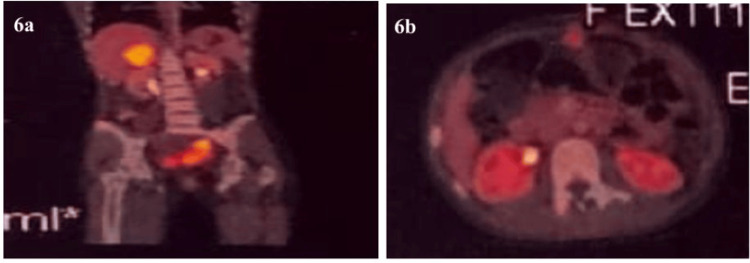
PET/CT images showing metastasis a: Sagittal view of active hypermetabolic areas of metastasis in the liver and spleen; b: Axial view of active hypermetabolic areas of metastasis in the right adrenal gland
